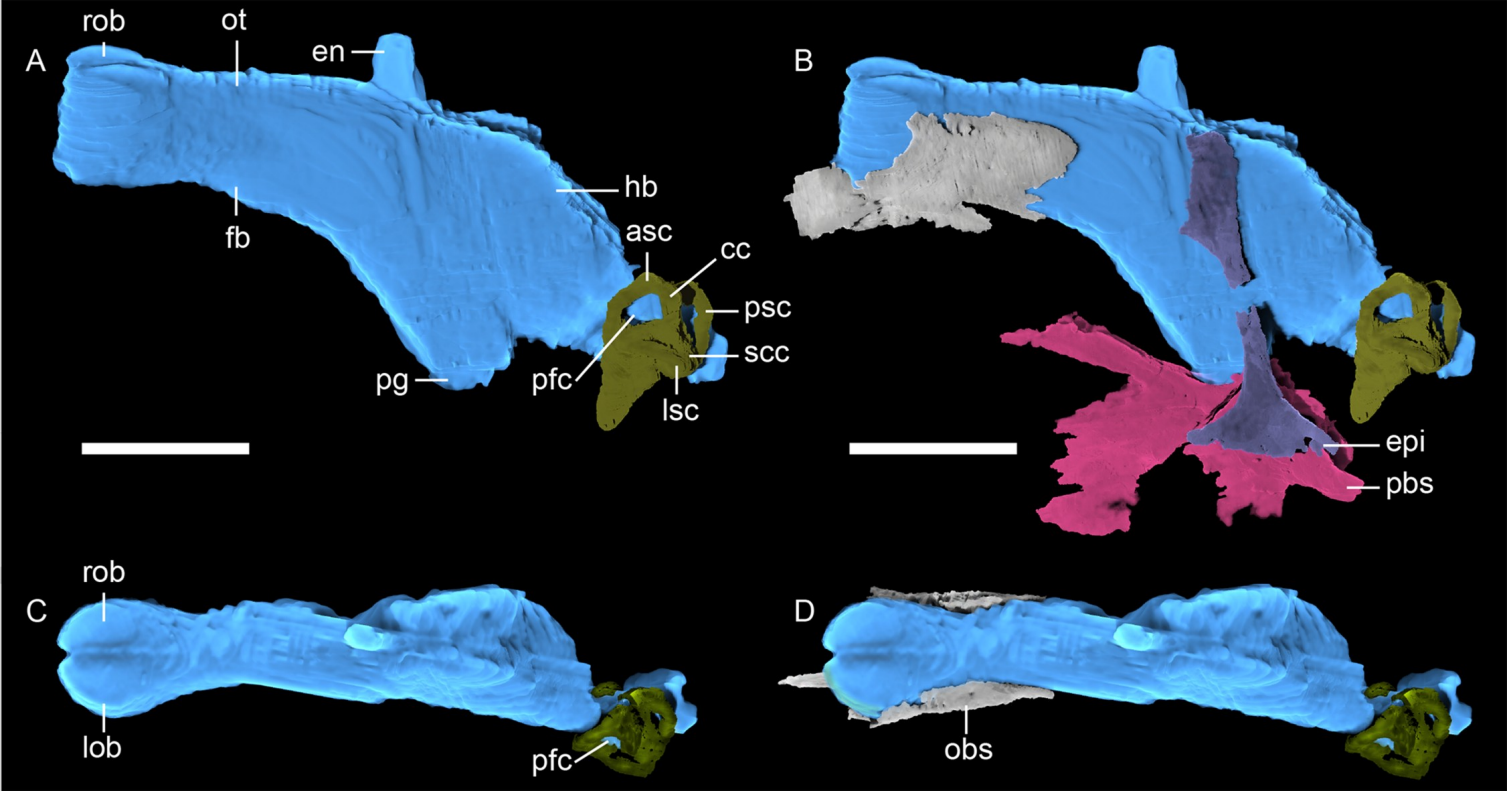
Neutron CT reconstruction of MB.R.999: brain endocast from (A and B) left lateral and (C and D) dorsal. Abbreviations: asc, anterior semicircular canal; cc, common crus; en, epiphyseal nerve; epi, epipterygoid; fb, forebrain; hb, hindbrain; lob, left olfactory bulb; ot, olfactory tract; pbs, parabasisphenoid; pfc, paraflocculus; pg, pituitary gland; psc, posterior semicircular canal; rob, right olfactory bulb; scc, secondary common crus. Scale bars = 2 cm.
